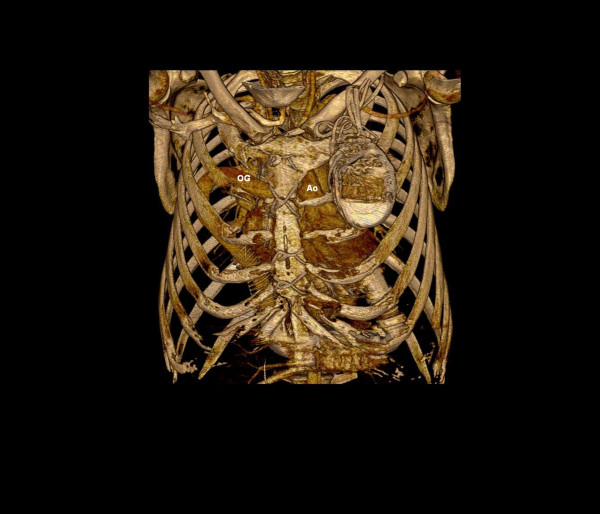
Reconstruction of CT images showing outflow graft (OG) passing behind the sternum before anastomosing aorta (Ao).
